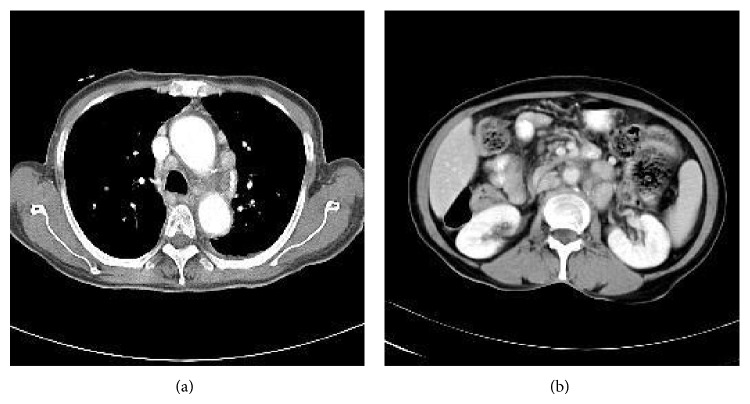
A chest CT (a) and an AP CT (b) show increased sizes of lymph nodes on mediastinum and retroperitoneal space after 12 months of follow-up loss.
